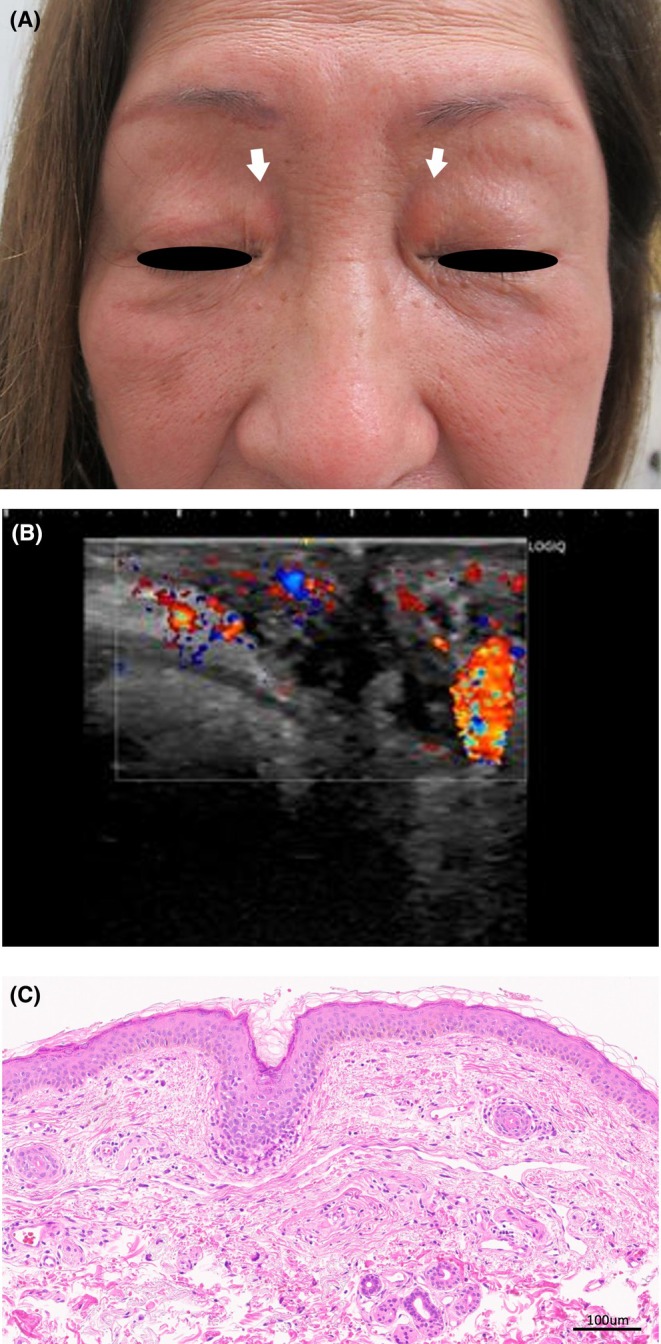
(A) A flat, erythematous, elevated lesion with well‐defined, elastic‐soft borders measuring approximately 10 mm in diameter on the medial aspect of the bilateral upper eyelids. No surface telangiectasia was observed. (B) Doppler skin ultrasonography revealed increased vascularity with a low‐flow pattern. (C) Skin biopsy of the upper eyelid lesion demonstrated diffuse vascular proliferation composed of slit‐like, irregularly shaped, thin‐walled vessels extending from the mid to deep dermis. The vessels exhibited mature luminal structures without endothelial cell atypia. No inflammatory cell infiltration, lipid deposition, or foam cells were present.
